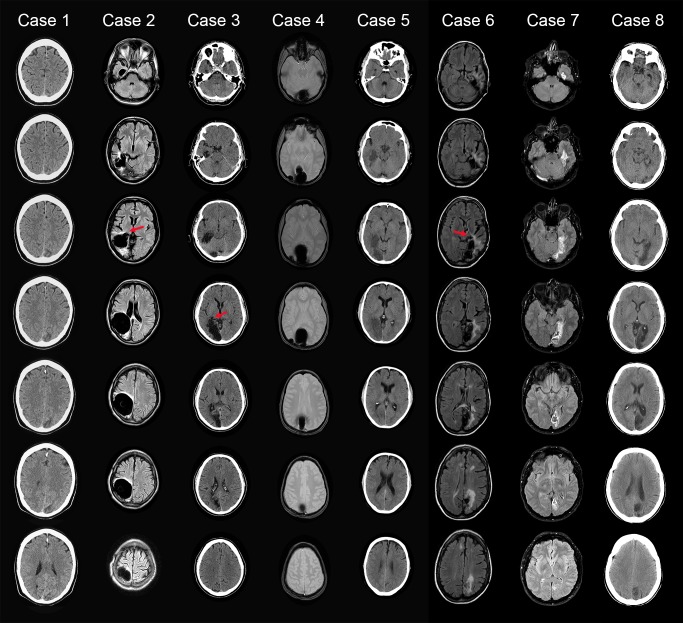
Axial images of the lesions of the participants. With CT for Cases 3, 5 and 8, MRI sequences for Cases 1, 2, 6, and 7 (FLAIR sequence) and Case 4 (SWI sequence). The arrows indicate possible LGN involvement. The left side of each slide represents the left side of the brain.
